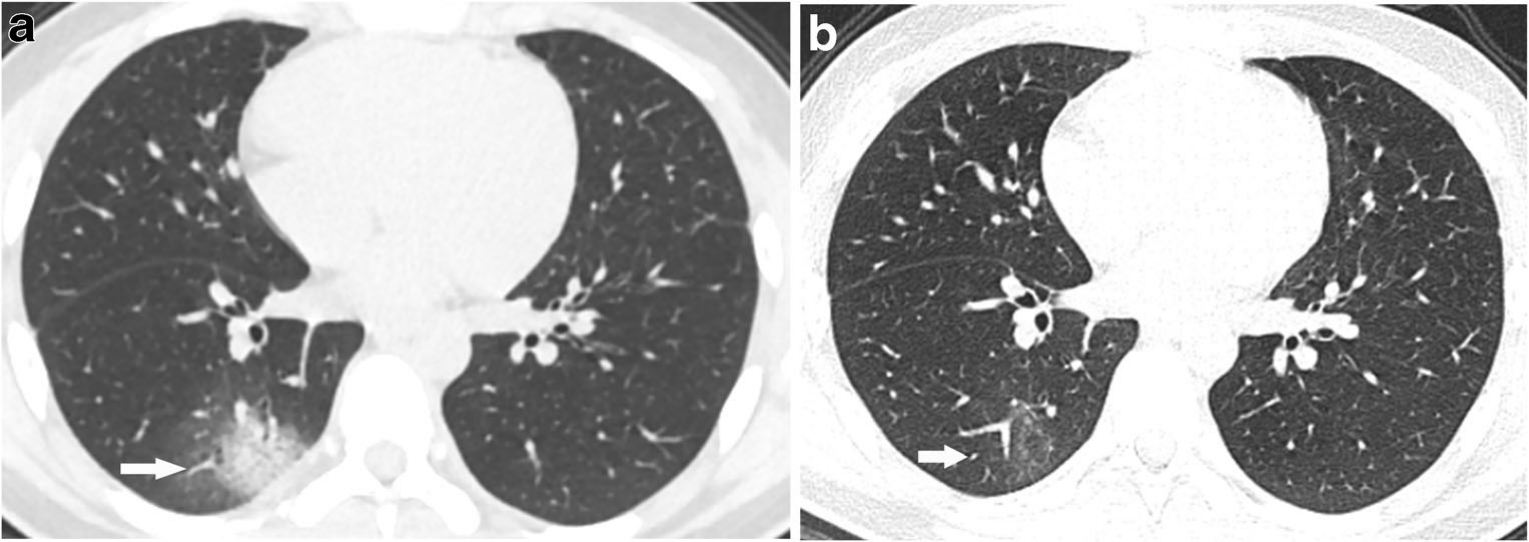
A 15-year-old asymptomatic boy with COVID-19 and a history of definite contact. a Axial thin-section non-contrast CT shows a subpleural rounded area of consolidation surrounded by ground glass, consistent with the halo sign and situated at the right lower lobe (*arrow*). b A follow-up CT image shows light ground-glass opacities after 9 days (*arrow*)
